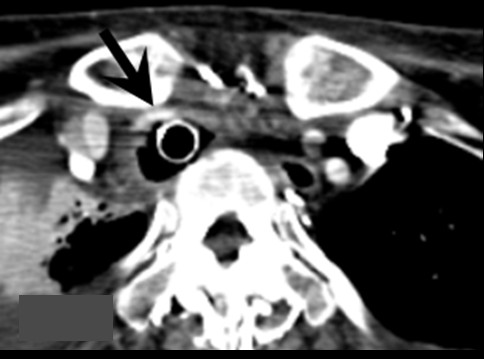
The narrowed brachiocephalic artery runs along and ventral to the tube (arrow). Although computed tomography detected no evidence of active bleeding, a tracheo-brachiocephalic artery fistula was suspected due to arterial bleeding.
